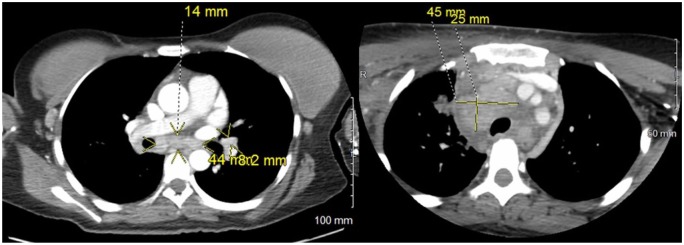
Computed tomography chest angiography to rule out pulmonary embolism instead
